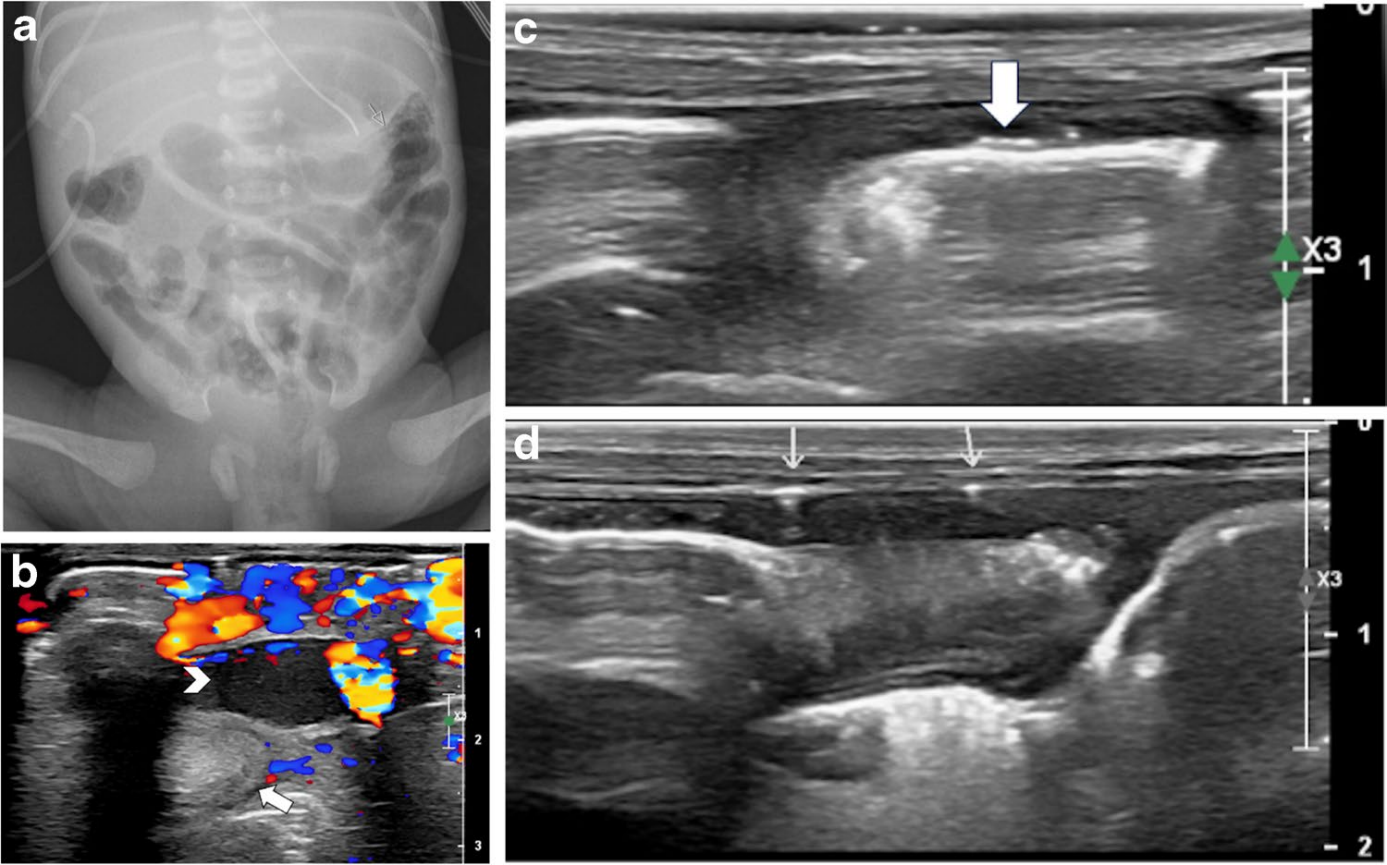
A 7-day-old preterm female born at 31 3/7 weeks (case 1 in Table 4). Abdominal ultrasound upgraded the modified Bell’s stage from 2 A to 3B. a An AP supine abdominal radiograph reveals pneumatosis in the right and left (*arrow*) upper quadrants. b Transverse abdominal ultrasound image of the right lower quadrant obtained 178 min later reveals bowel hypervascularity, bowel wall thickening (*arrow*), and complex interloop fluid (*arrowhead*). c Transverse ultrasound image of the right upper quadrant demonstrates gas within the bowel wall (*arrow*) with intraluminal gas deep to it. d Transverse ultrasound image of the right upper quadrant demonstrates small volume pneumoperitoneum (*arrows*) within complex free fluid
